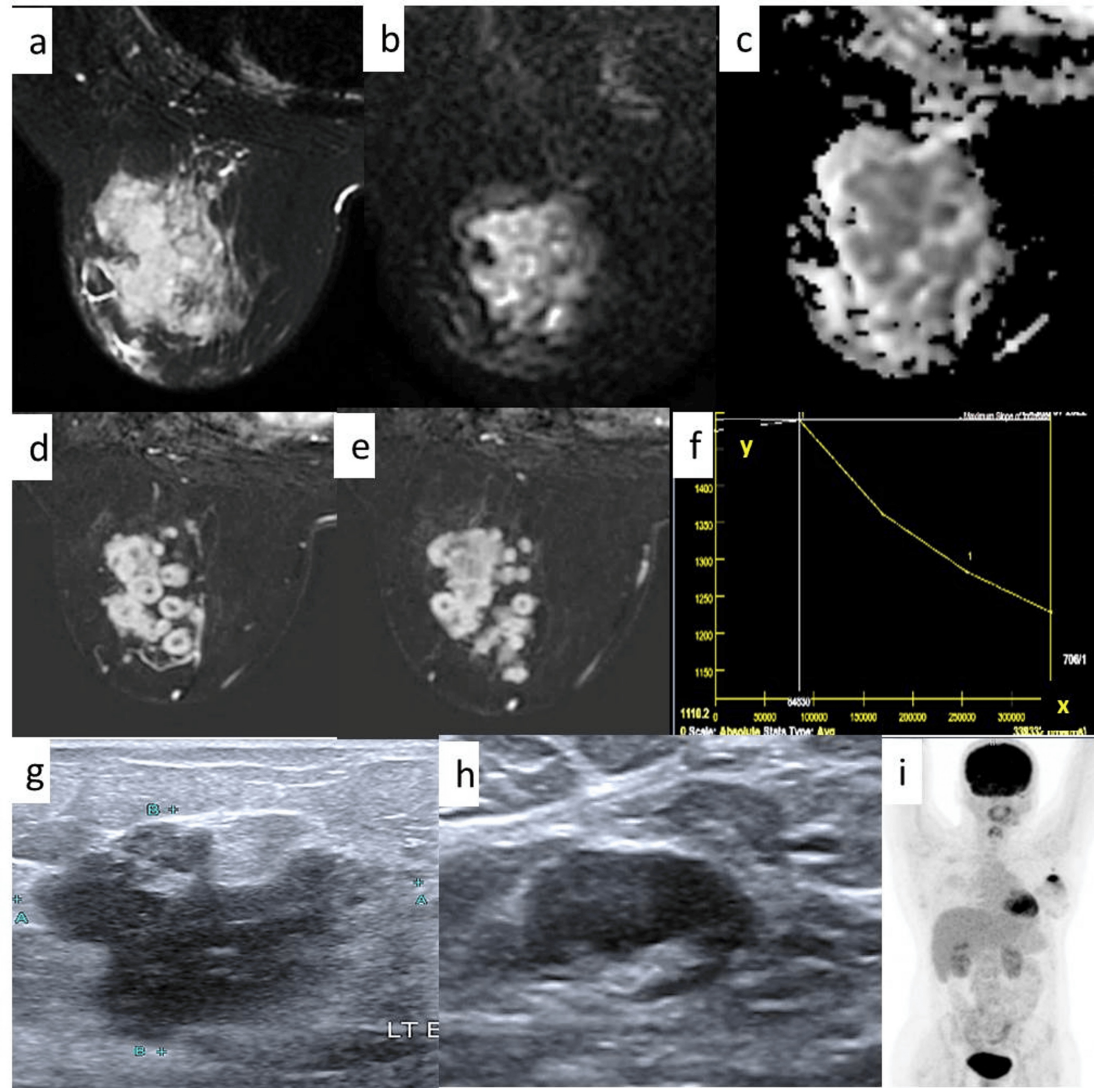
A case of a 23-year-old female a-c: T2-weighted, DWI, and ADC images show a T2 isointense non-mass area with diffusion restriction and a corresponding drop in signal in ADC; d,e: MRI dynamic contrast images show non-mass clumped ring enhancement in diffuse distribution; f: MRI shows a time intensity curve with a type 3 kinetic curve, with the x and y axis representing the time and signal intensity, respectively; g,h: ultrasound shows an irregular hypoechoic mass and a lymph node with cortical thickening in the ipsilateral axilla; i: FDG PET scout image shows no distant metastases. DWI: diffusion weighted imaging; ADC: apparent diffusion coefficient; FDG: fluorodeoxyglucose
